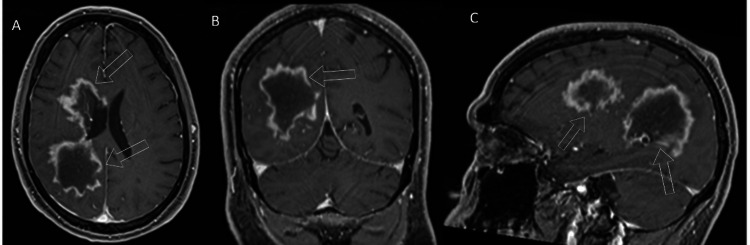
Initial MRI on presentation Enhanced MRI demonstrates mass-like lesions with a T1 hypointense signal relative to unaffected white matter with avid peripheral enhancement as marked by the unfilled white arrows. The provided views included axial (A), coronal (B), and sagittal (C). The enhancement pattern is frondlike and slightly discontinuous.
